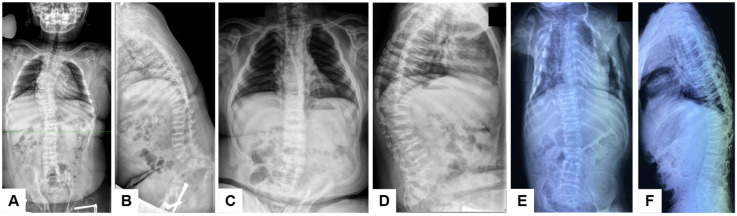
Anteroposterior and lateral radiographs of the spine in patients P1–P3. P1, 13 years (A,B): right-sided scoliosis of 40 degrees, kyphosis of 33 degrees, platyspondyly, and sacrococcygeal abnormalities. P2, 10 years (C,D): localized pathological thoracic kyphosis of 45 degrees with reduced vertebral body height and vertebral wedging, lumbar hyperlordosis, and a left-sided thoracolumbar scoliotic curve of 26 degrees. P3, 10 years (E,F): sequelae of multiple rib fractures with callus formation, pseudo-butterfly configuration of the biconcave vertebral bodies, S-shaped clavicular deformities, narrowing of the upper thorax, and asymmetric platyspondyly with anterior wedging and secondary kyphosis.
